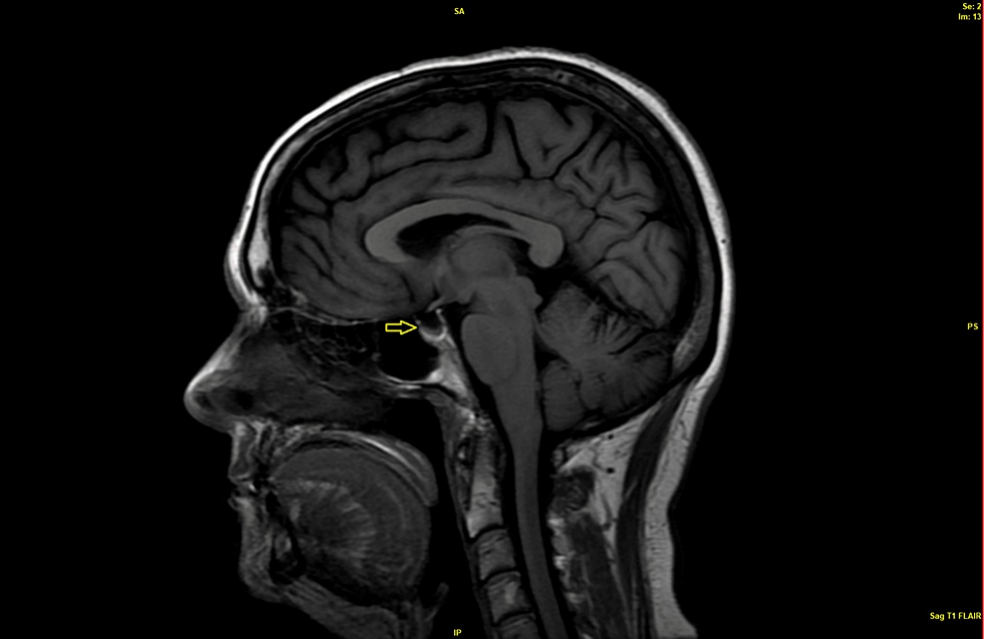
Brain MRI, sagittal view with partial empty sella
